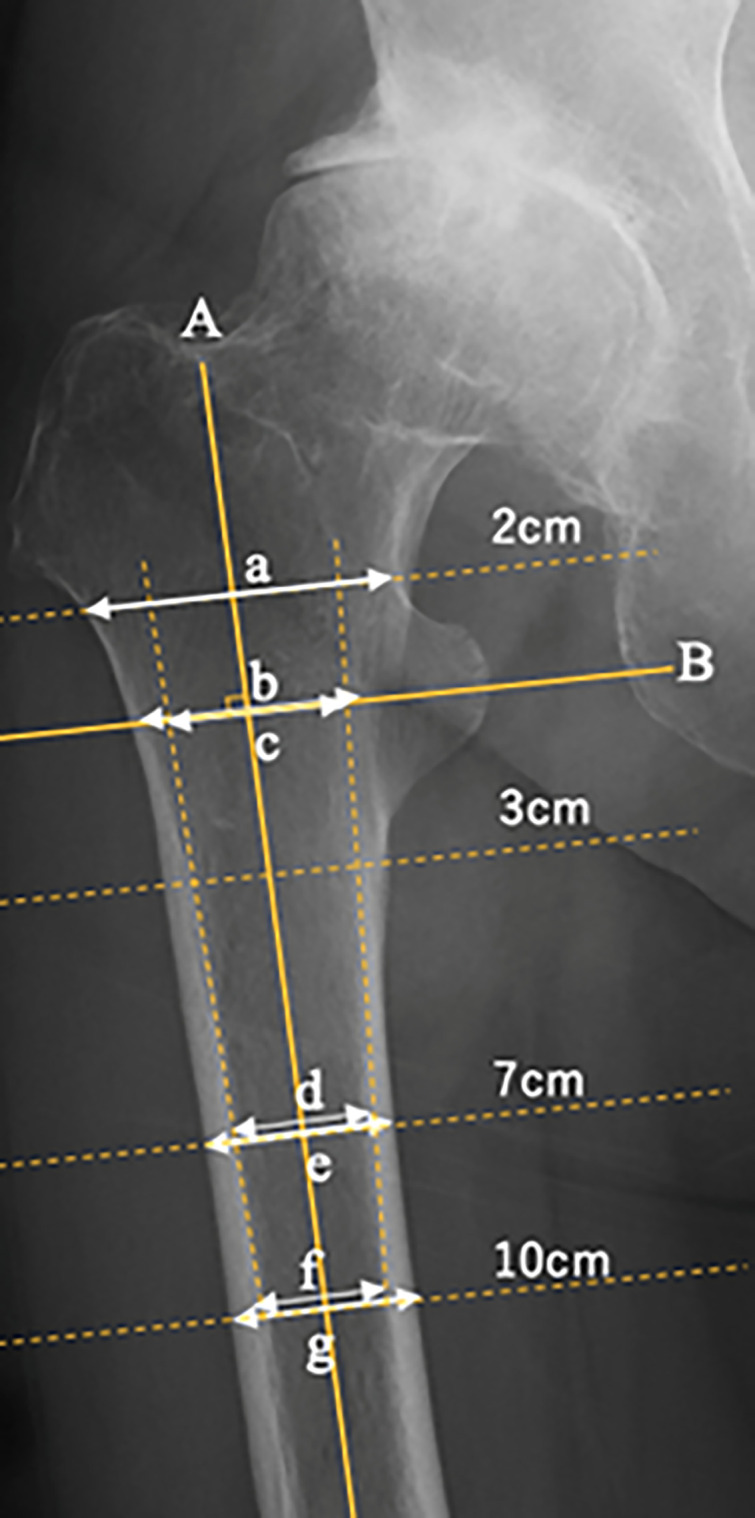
Proximal femoral morphological indices. Radiographic femoral indices included canal-to-calcar ratio (CCR): f/b; canal diaphysis ratio (CDR): d/e; canal flair index (CFI): a/f; cortical thickness index (CTI): f/g; canal bone area ratio (CBAR): (d + f)/ (e + g); Line A: femoral axis; Line B: perpendicular to femoral axis at lesser trochanter midpoint.
